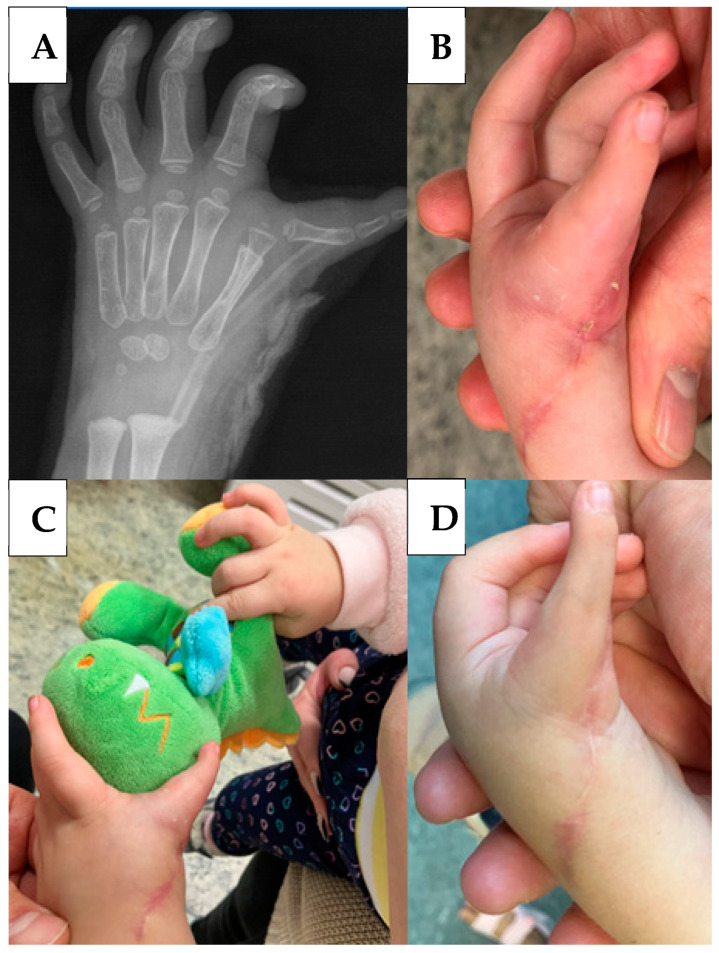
Control X-ray in the fourth week. The metacarpus of the preaxial I wedged into the metacarpus of the preaxial M can be visualised (A). The surgical wound (B) and the grasping movement (C) in the second month. The surgical site sixth months post surgery (D).
